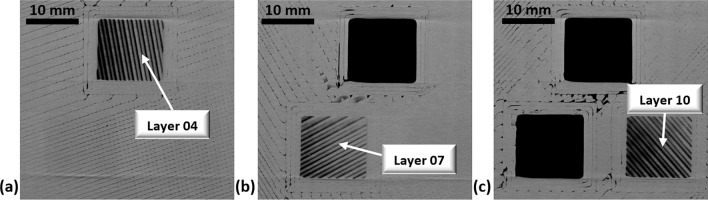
Extracted images of Layer 04, 07 and 10 at their individual depths using X-ray CT.
